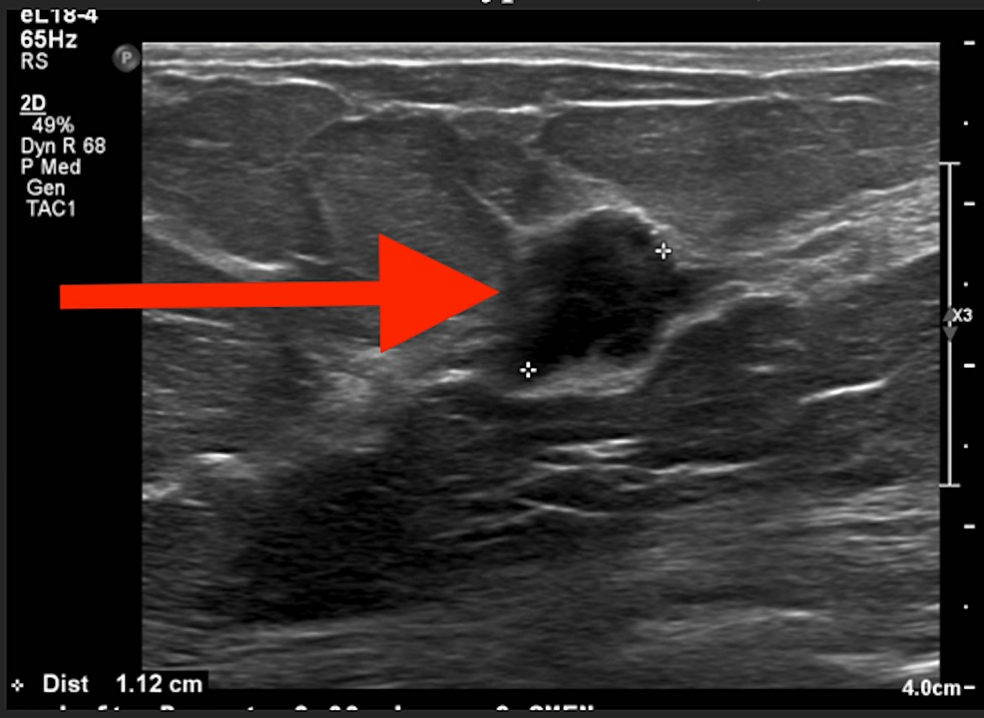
Ultrasound of the left breast showing a hypoechoic mass, measuring 1.1 cm in diameter, with irregular borders.
